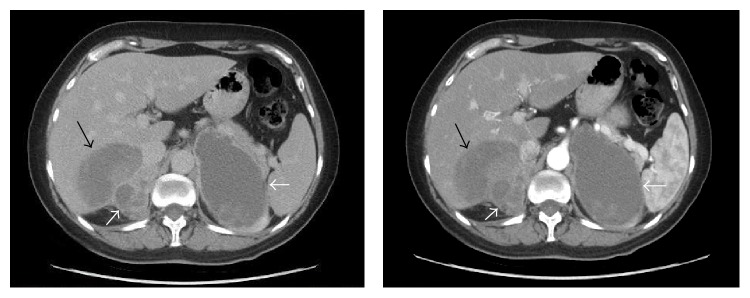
Computed tomography (CT) of abdomen; cystic masses replacing both adrenal glands and infiltrating liver can be seen (arrows).
